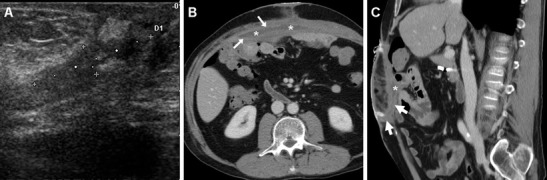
A 51-year-old male experienced wound dehiscence with culture-proven *Staphylococcus aureus* infection following Rives-Stoppa repair of a ventral incisional hernia. Ultrasound (a) showed an ill-defined, inhomogeneously hypoechoic region (caliper) at the surgical site, without fluid portions amenable to aspiration. Corresponding post-contrast axial (b) and sagittal (c) CT images showed a band-shaped abscess (*) of the anterior abdominal wall with enhancing periphery (arrows), interposed between the PM and rectus muscles, which drained externally through a fistula (thick arrows in c) (note hyperattenuating plug at skin orifice)
